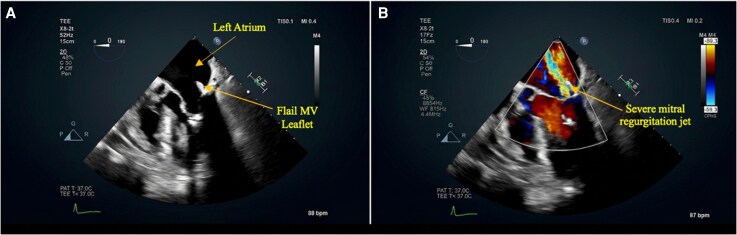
Transoesophageal echocardiographic assessment of acute mechanical valvular failure. (*A*) 3D transoesophageal echocardiogram (mid-oesophageal view) demonstrating acute structural collapse of the subvalvular apparatus. The arrowhead highlights a distinct flail posterior mitral valve leaflet prolapsing retrogradely into a dilated left atrium, secondary to an acute rupture of the posteromedial papillary muscle head. (*B*) Transoesophageal echocardiogram with colour Doppler evaluation over the identical mid-oesophageal plane. The colour flow mapping reveals a massive, highly eccentric, high-velocity jet filling much of the left atrium, confirming severe (4+) acute mitral regurgitation resulting from the subvalvular mechanical failure.
